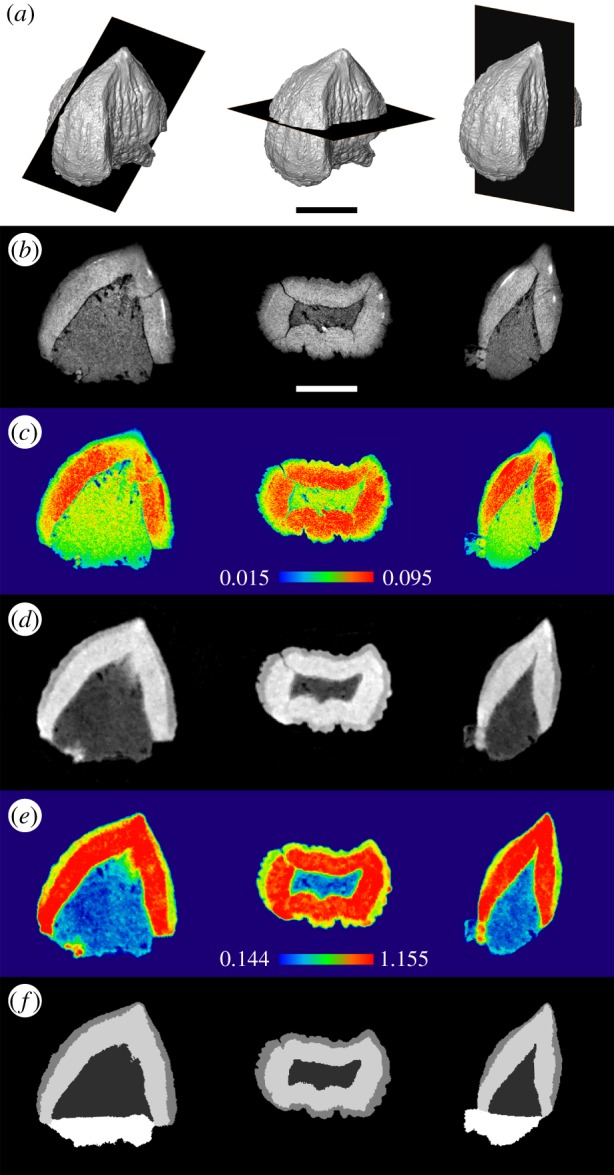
Sections of the left posteriormost tooth of *Eilenodon* (DMNH EPV.10685) made using X-ray CT and neutron tomography. (*a*) Computer models showing the location of the coronal, horizontal and mesiodistal section. (*b*) Final segmented model based on neutron attenuation. (*c*) X-ray results. (*d*) Neutron results. (*e*) X-ray results coloured according to attenuation. (*f*) Neutron results artificially coloured according to attenuation. Scale bar = 2 mm.
